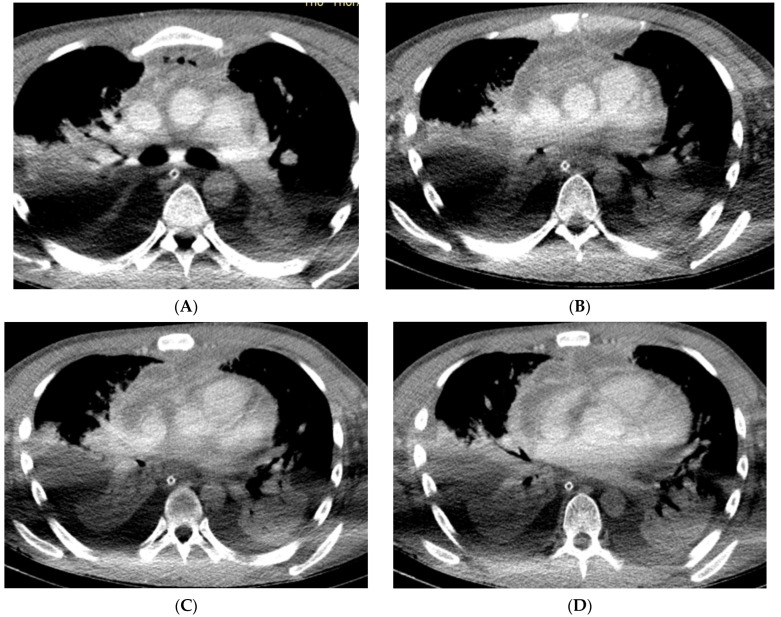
(Patient 4) Axial contrast-enhanced CT image; (A–D) mediastinal empyema on the anterior mediastinum and retrosternal space and bilateral pleural effusions.
